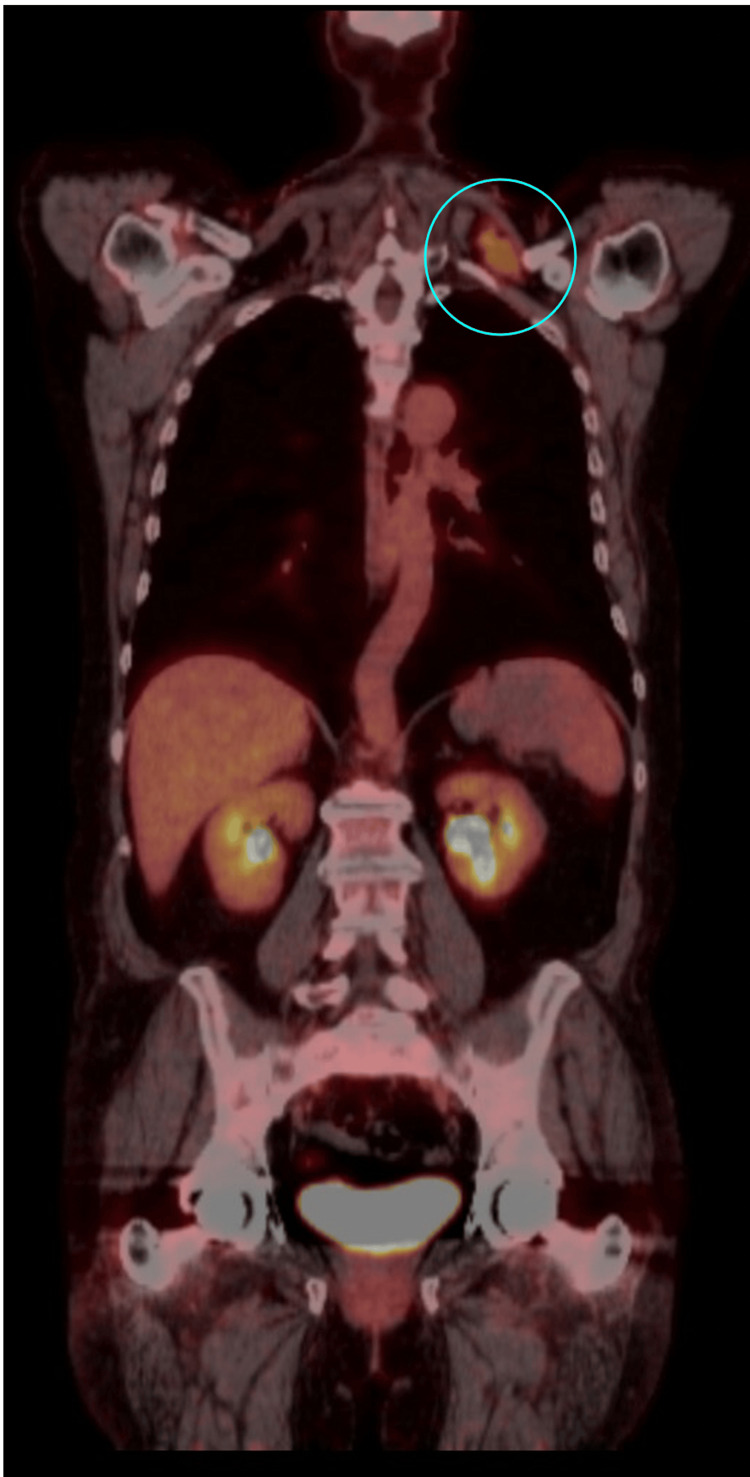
PET-CT scan from skull to thigh, coronal view, with a cyan circle marking a solitary FDG-avid left supraclavicular lymph node, measuring 3.6 x 2.4 cm with an SUV max of 4.71. No other suspicious FDG-avid lesions are observed. PET-CT: positron emission tomography-computed tomography; FDG: fluorodeoxyglucose; SUV: standardized uptake value
